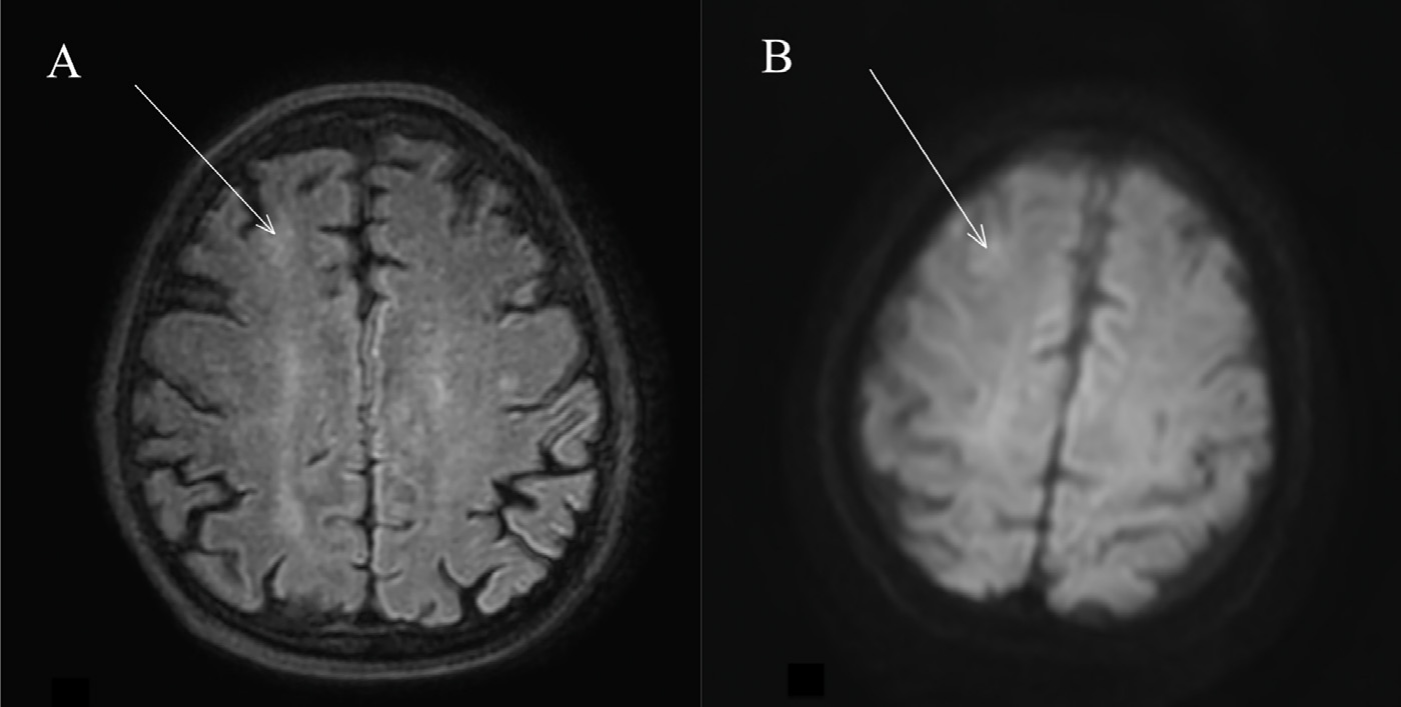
Fluid attenuated inversion recovery (FLAIR) and diffusion-weighted-imaging (DWI) in (A) and in (B). Areas of vague hyperintense signal alteration (white arrows in A and B) due to sub-acute ischemic lesions.
